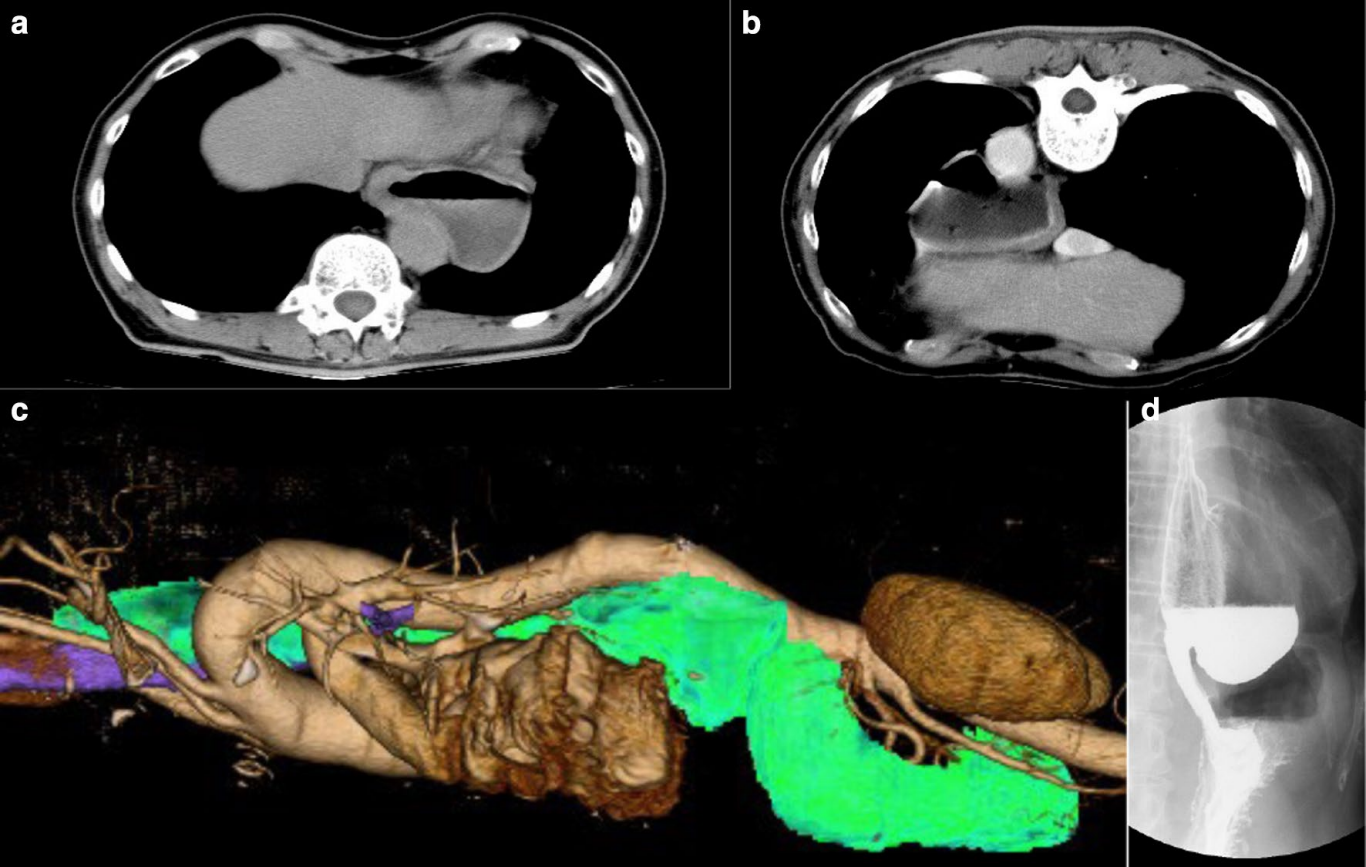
Preoperative CT and esophagogram. a CT in the supine position. b CT in the prone position. c 3D-CT of the epiphrenic diverticulum. d: Esophagogram
